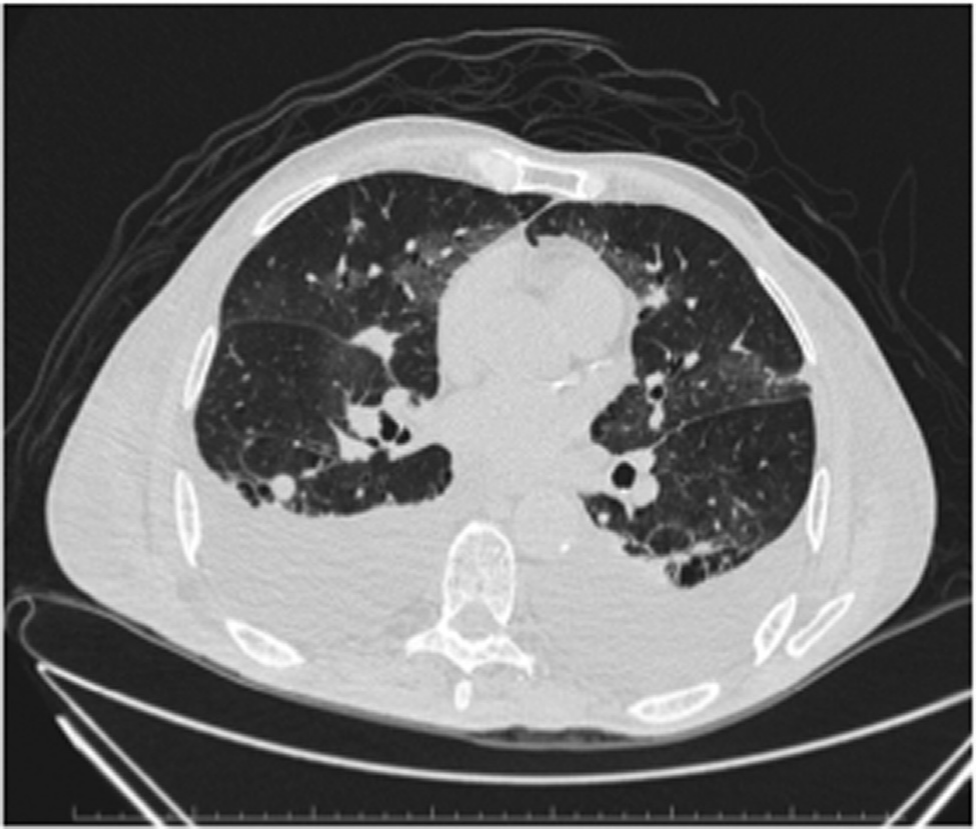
Chest computed tomography scan. In July 2016, the patient had pleural effusion and multiple metastatic nodules in both lungs and died in August 2016.
